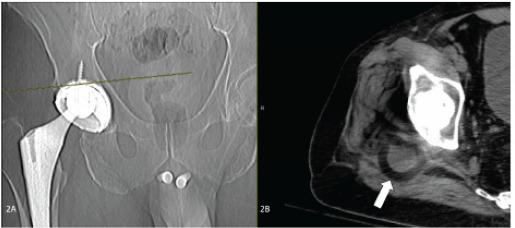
Computed tomography post reduction scout film (2A) and representative axial slice (2B) showing reduction of the femoral component into the acetabular cup, but with persistent intraprosthetic dislocation of the acetabular liner (arrow).
